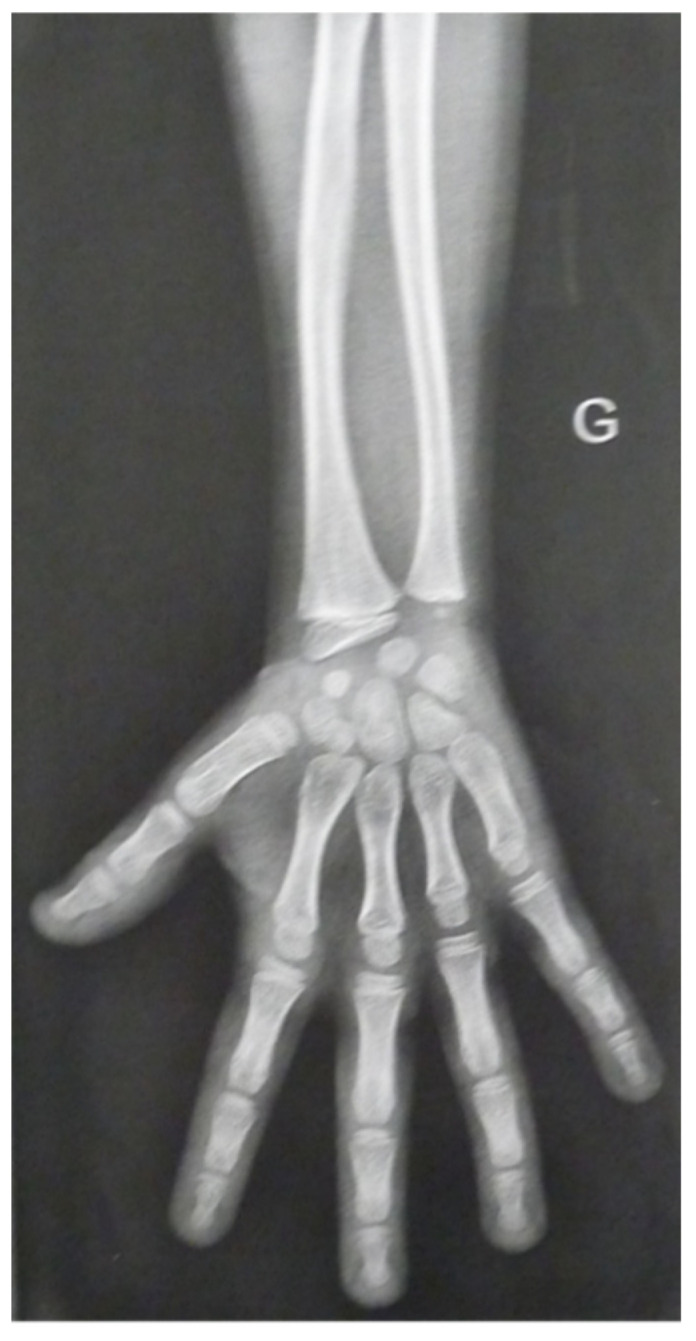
Radiograph at 7 years and 5 months of the left forearm of individual IV.3 showing slight brachymetacarpy of the 4th and 5th fingers. G is for “gauche”, it is “left” in french.
